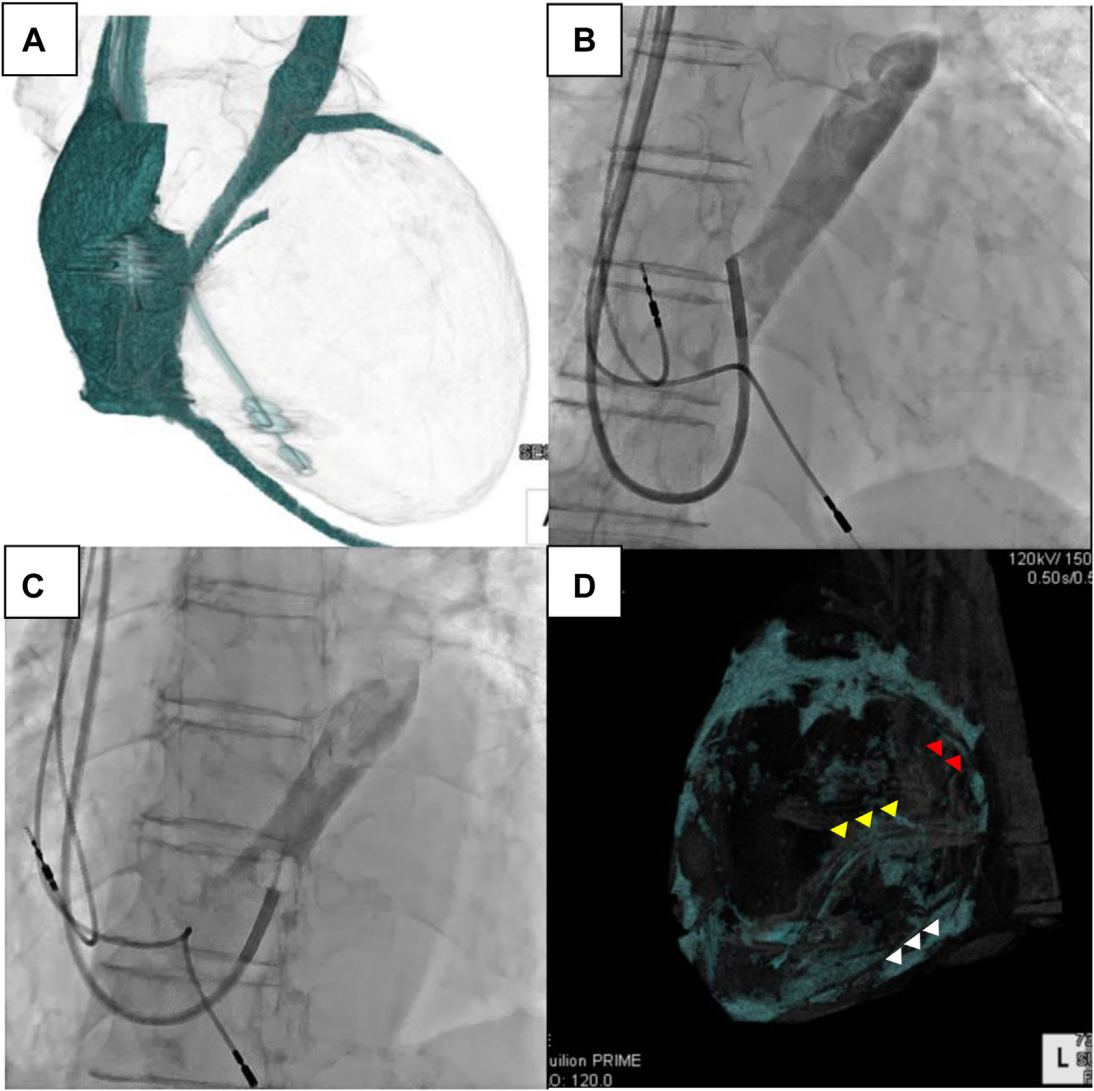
A: Contrast cardiac computed tomography (CT) image of anterior-posterior view showing persistent left superior vena cava and a coronary sinus (CS) without a lateral vein. CS branches are manually traced on a 2D image and reconstructed to a 3D image. B: Venography from the mid CS trunk. C: Occlusive venogram failed to visualize the CS branch. D: Noncontrast cardiac CT image using the fat description method of the left posterolateral view. Red arrowheads show the anterior intraventricular vein orifice. Yellow arrowheads show the right ventricular lead. White arrowheads show the middle cardiac vein.
